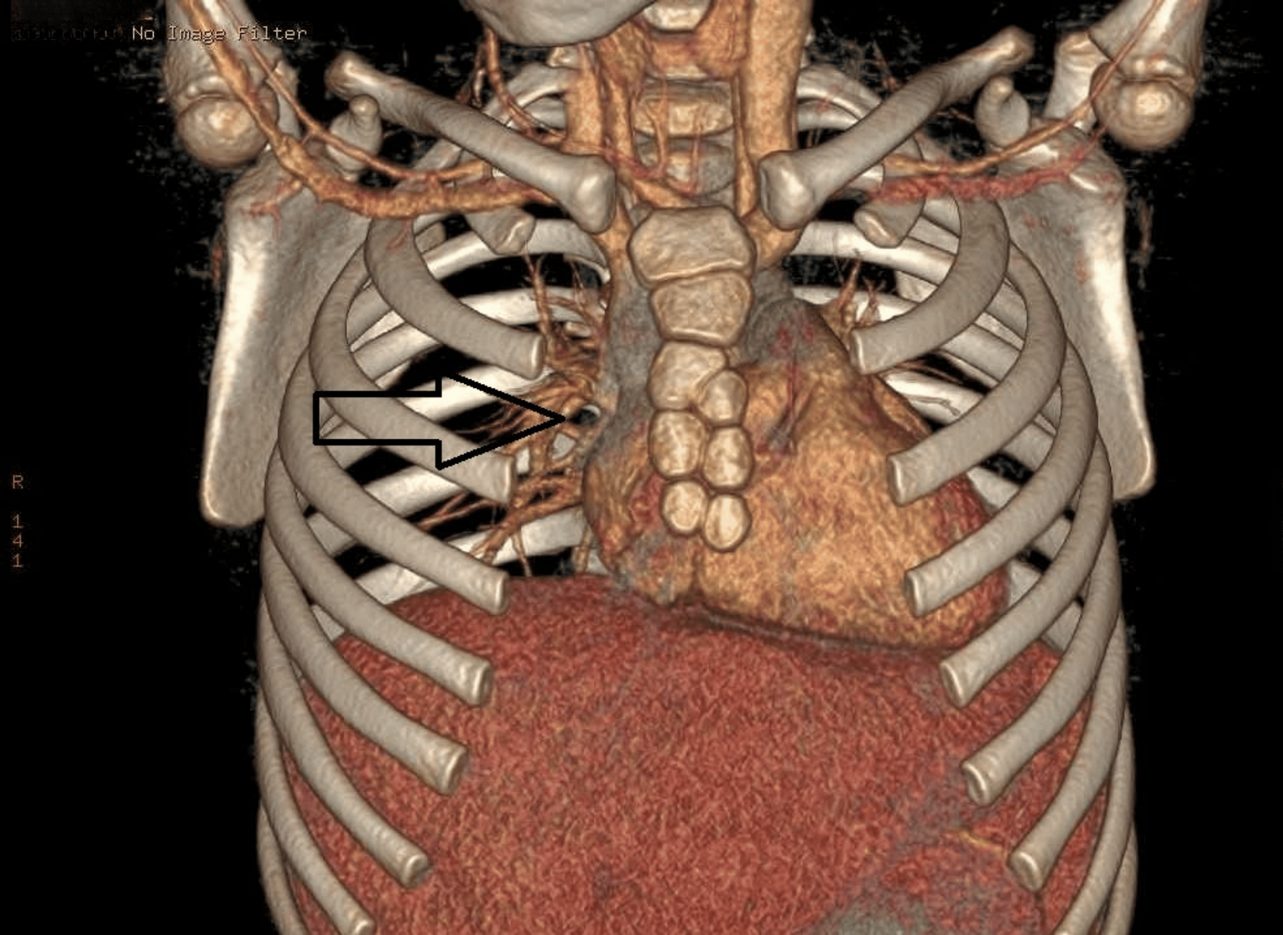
CT 3D deconstruction image showing the honeycomb appearance of the sternum CT: computed tomography, 3D: three dimensional
